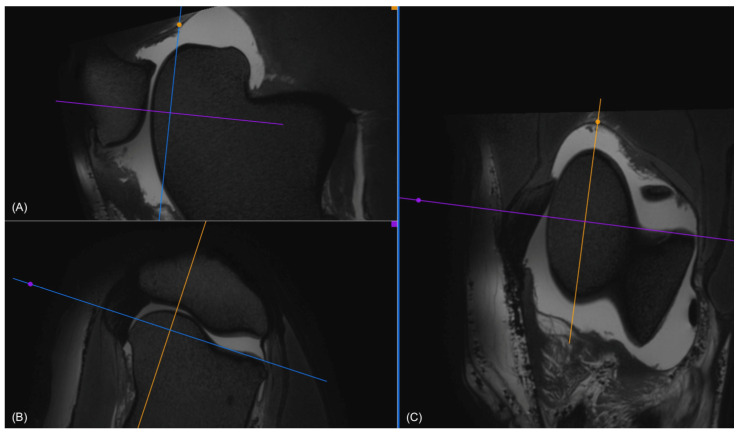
(A) Sagittal, (B) transverse, and (C) dorsal view in 3D VIEW T2-weighted images of a stifle, where the regions of interest (ROIs) were determined for the medial trochlear ridge. In the transverse view (B), the dorsal plane (blue) was oriented to be parallel to the joint space. In the dorsal view (C), the sagittal plane (yellow) was oriented according to the long axis of the medial ridge. This was repeated for lateral and axial measurements by orienting the sagittal plane (yellow), according to the long axis of the lateral ridge and the trochlear groove, respectively.
